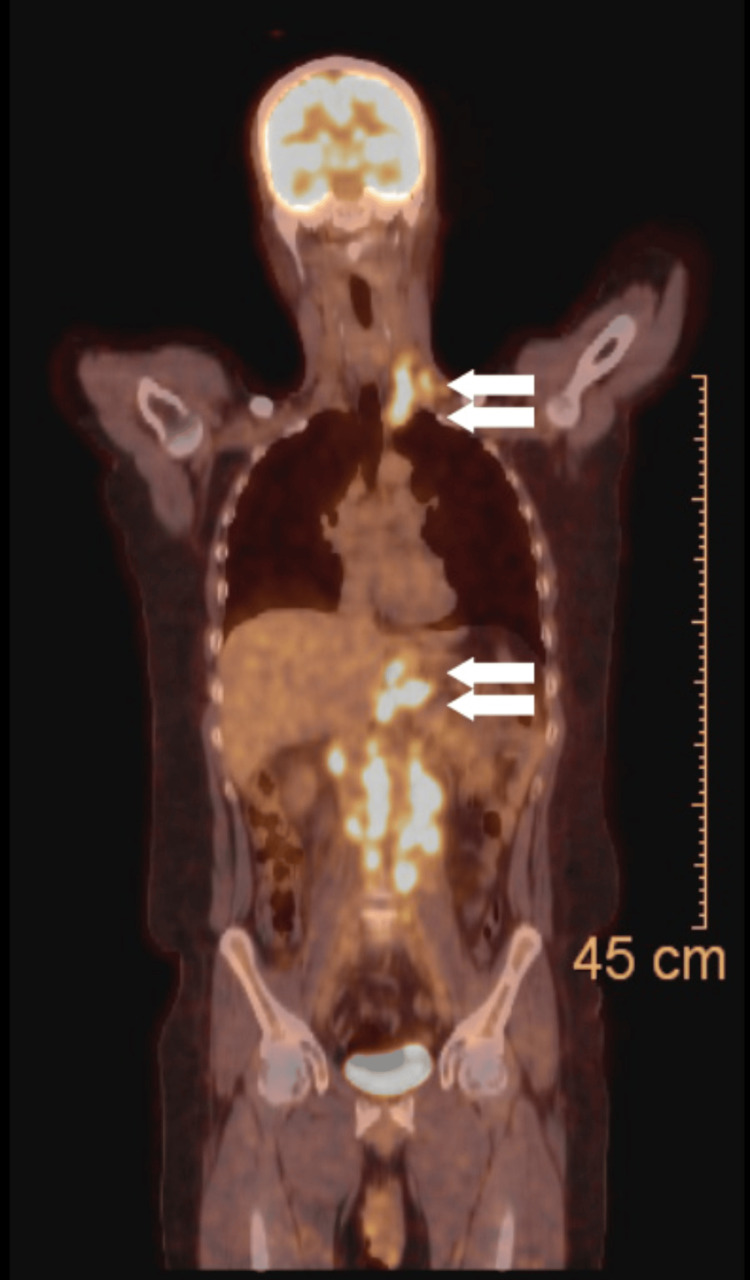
Positron emission tomography-computed tomography scan showing hypermetabolic activity at the gastric fundus extending to the gastroesophageal junction and the distal end of the esophagus and multiple enlarged hypermetabolic metastatic lymph nodes in the left lower neck and mediastinal paraesophageal lymph nodes.
